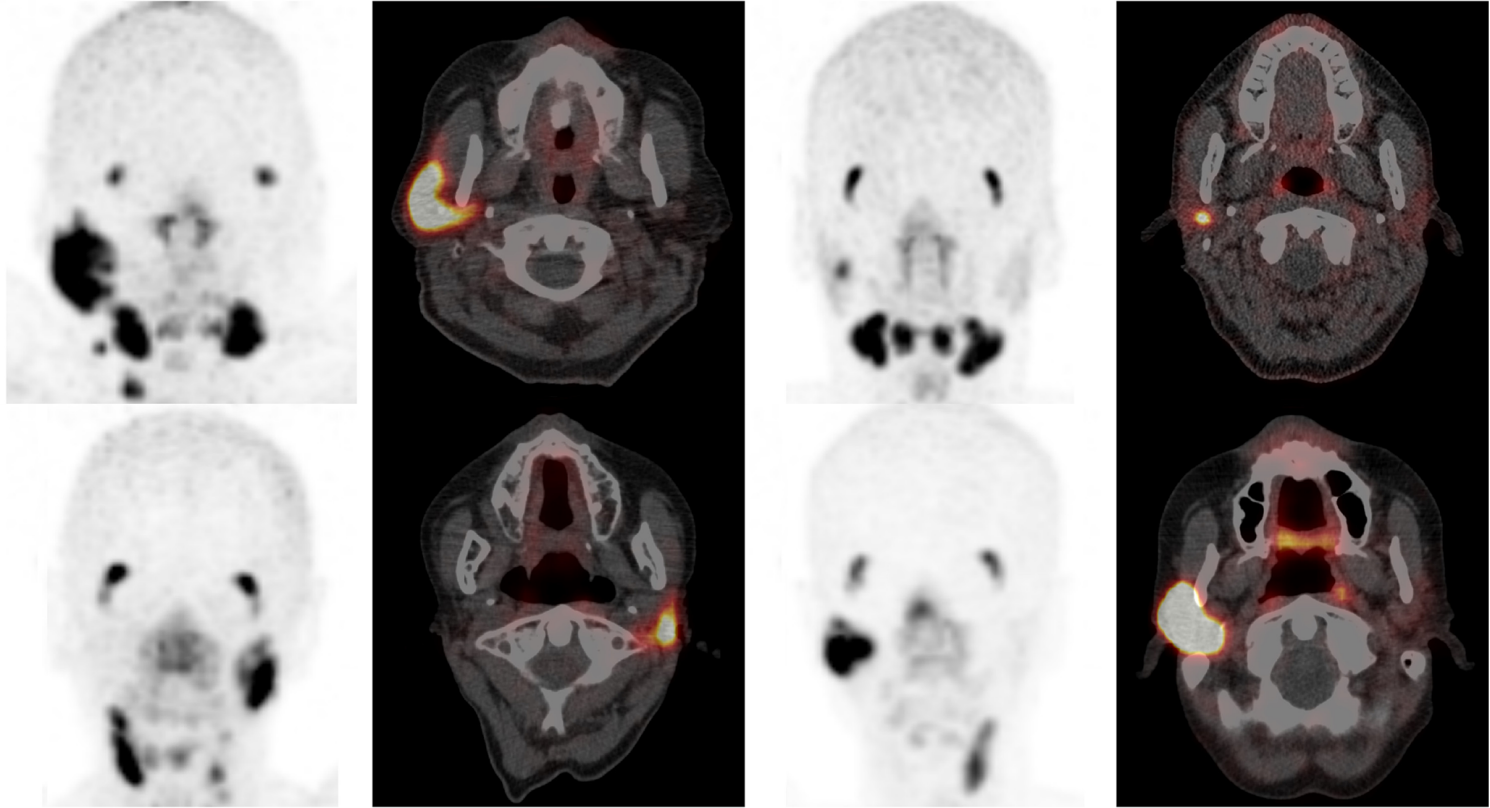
^68^Ga-PSMA PET/CT images acquired for 4 patients with a history of multiple ^131^I therapy cycles. This image was reproduced from Mohan et al. with permission [109]
